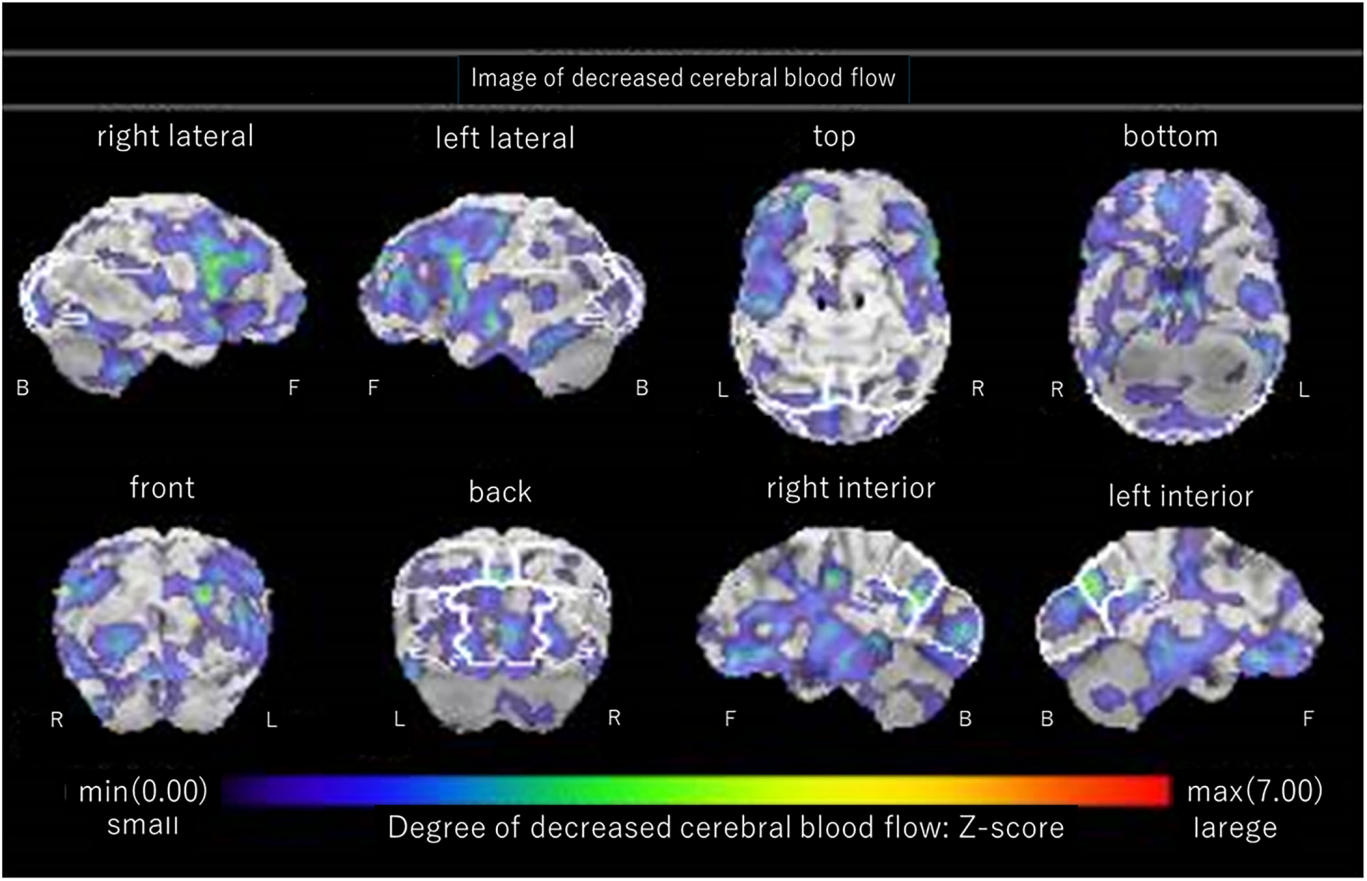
Single photon emission computed tomography on the 42nd day in the hospital indicating indicated reduced blood flow in both frontal lobes.
